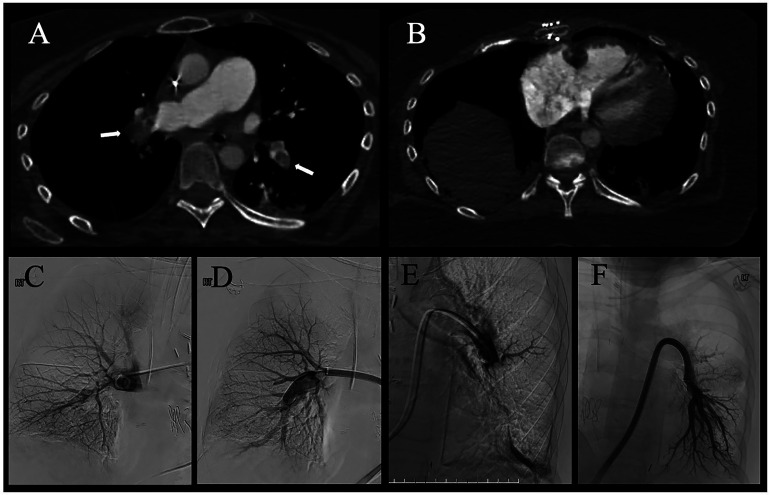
Computed tomography pulmonary angiogram and catheter pulmonary angiograms. (A) Extensive burden of pulmonary embolism bilaterally in the right and left pulmonary arteries extending into the lower lobes (arrows) with the right pulmonary vascular anastomosis seen, (B) enlarged right ventricle with increased RV:LV ratio and flattening of the interventricular septum. Right (C) and left (E) catheter pulmonary angiograms before LBMT demonstrating multiple filling defects, which are acute emboli and reduced pulmonary perfusion. Right (D) and left (F) catheter pulmonary angiograms after LBMT with resolution of the emboli and restoration of flow into the lower lobe vessels bilaterally.
